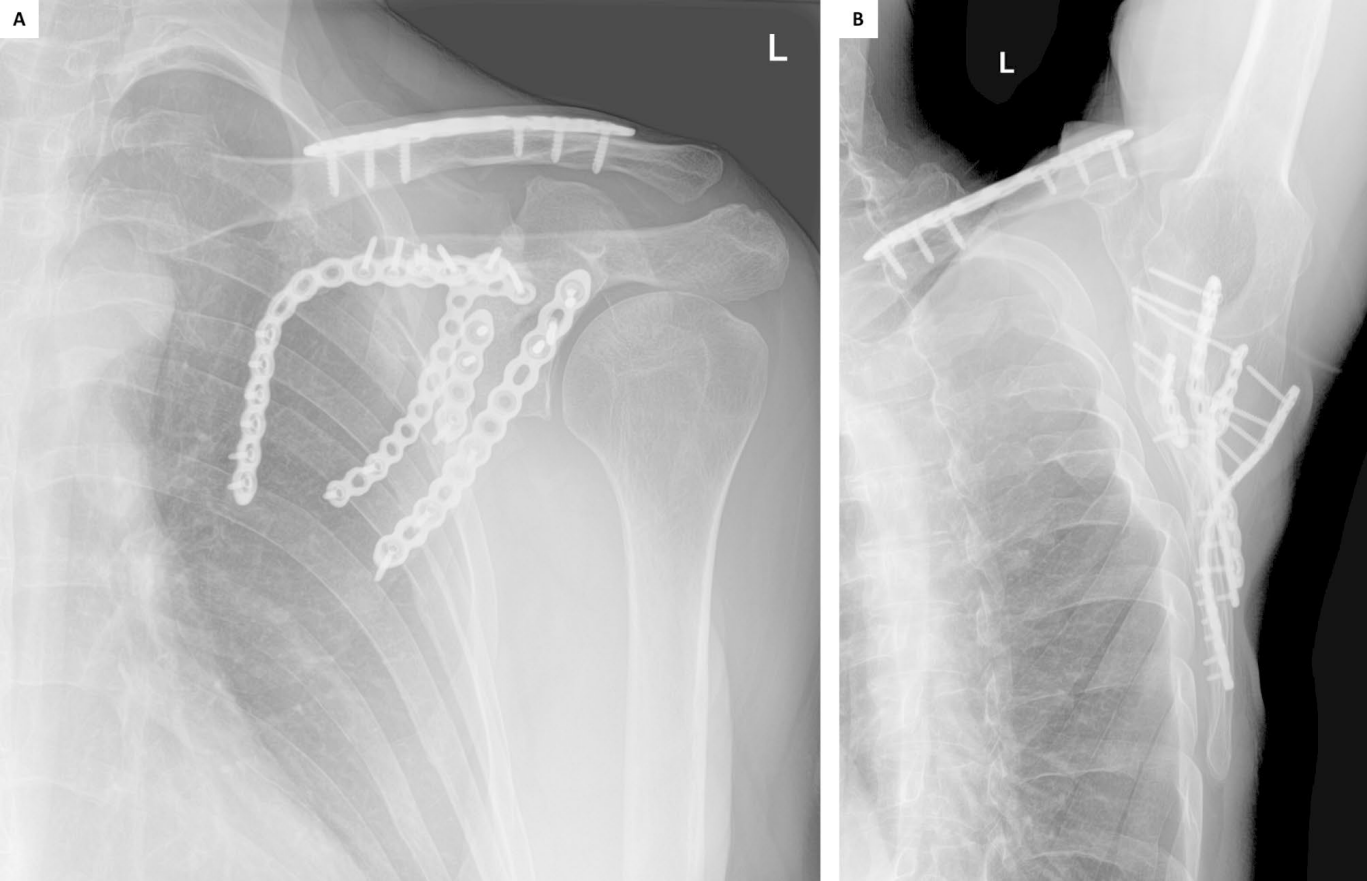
X-ray images of the left scapula 3 months after surgery. A: Grashey view. B: axillary Y view
